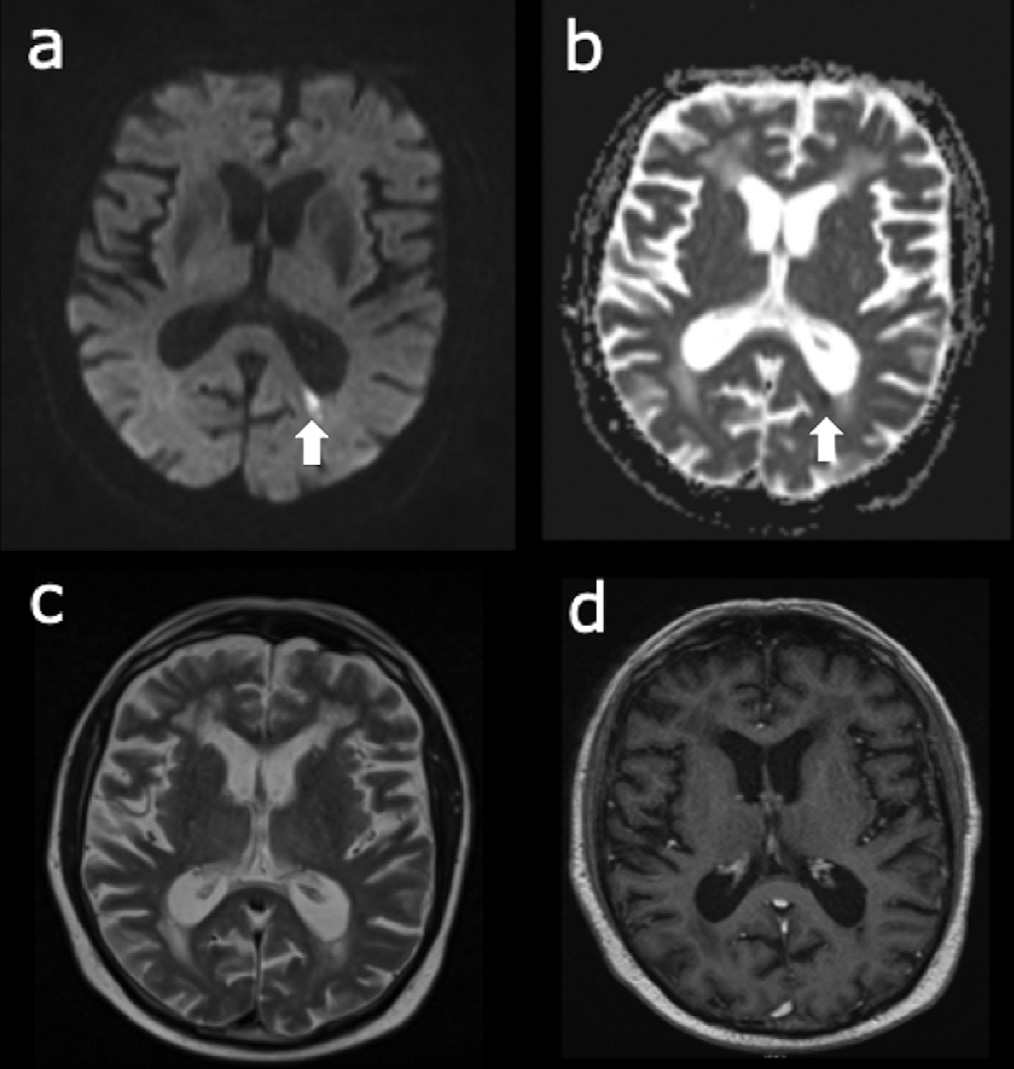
Repeat MRI images under sedation (a) DWI image showing a small left occipital lesion (arrow); (b) ADC map showing a small area of low ADC (arrow) corresponding to the lesion on DWI; (c) *T*_2_ weighted image showing no significant changes in the region of the lesion; (d) post-contrast image showing no contrast uptake or ring-enhancement at the site of the lesion. ADC, apparent diffusion coefficient; DWI, diffusion-weighted imaging.
